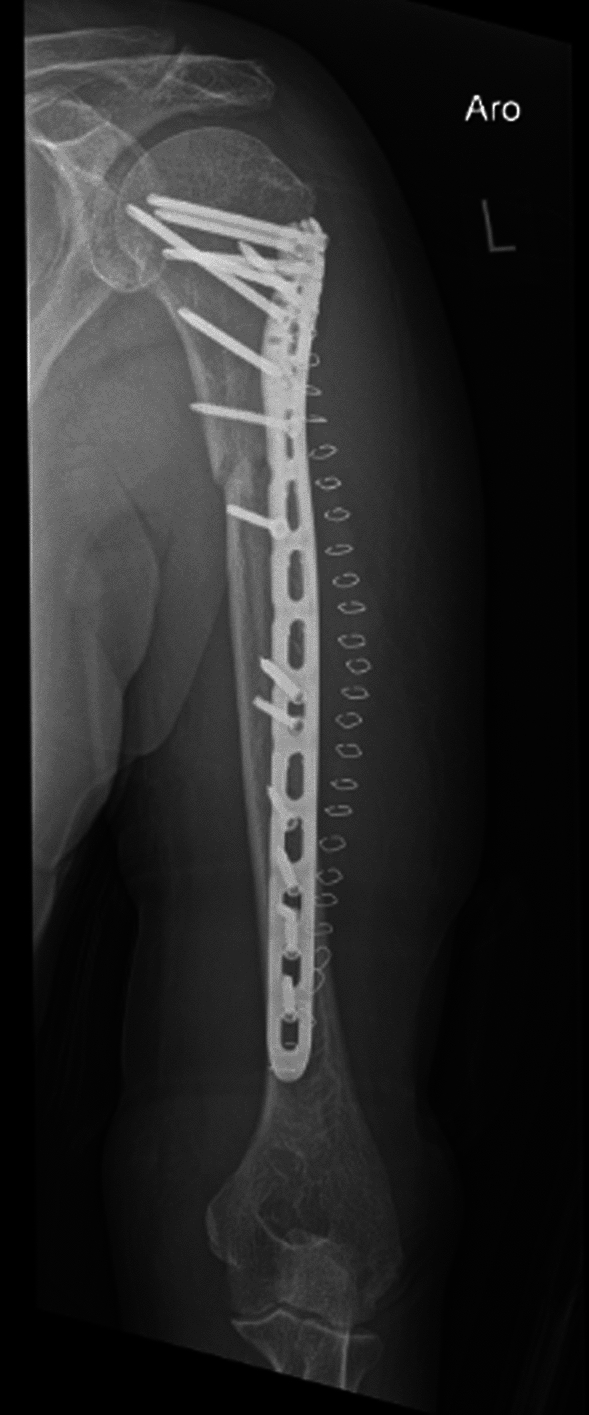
X-ray in external rotation two days after ORIF with a twisted 10 holes-Shaft Long PHILOS^®^ Plate (Synthes, Oberdorf, Switzerland) after proximal humerus shaft fracture [12]. Exact knowledge of the radial nerve’s location before surgery of proximal humerus shaft fractures is essential and can be optimized with ultrasound on the affected or contralateral side
